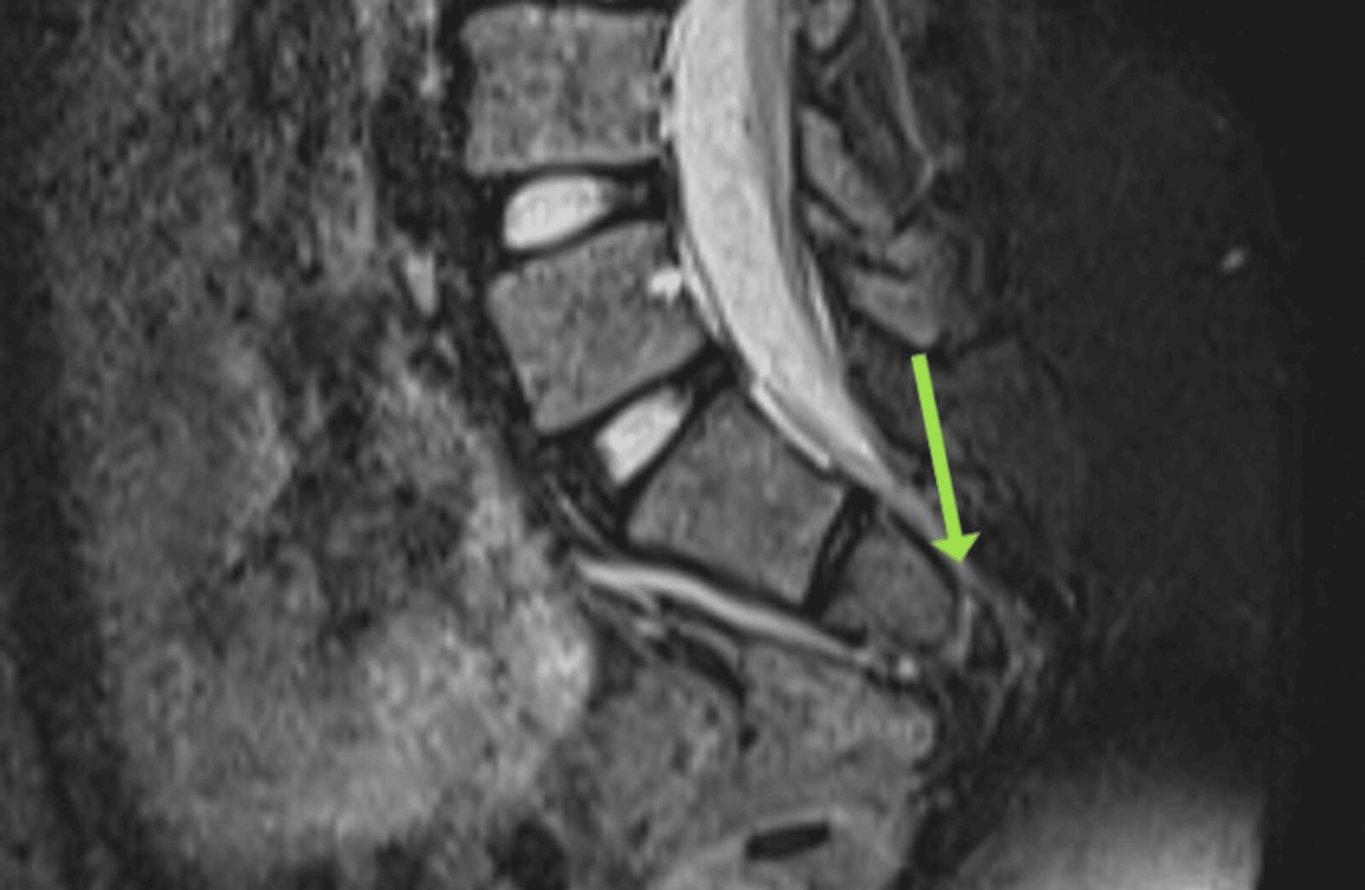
MRI image The arrow shows caudal agenesis.
